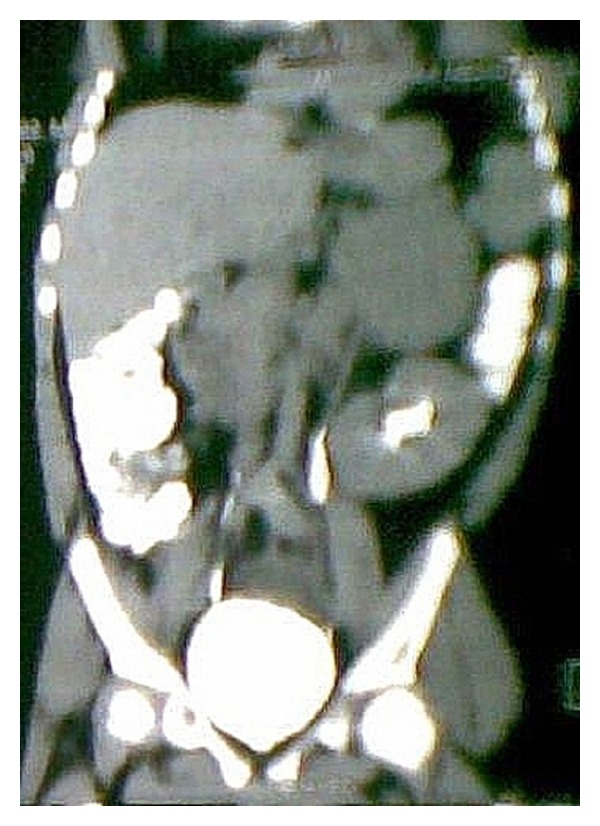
Contrast-enhanced abdominal computed tomography (CECT) scan in axial view revealed a large, well-circumscribed mildly enhancing adrenal mass (8 × 6 × 5 cm approx.) having few internal calcifications at left suprarenal region, which had displaced left kidney slightly downwards (arrow-mass; triangle-left kidney).
